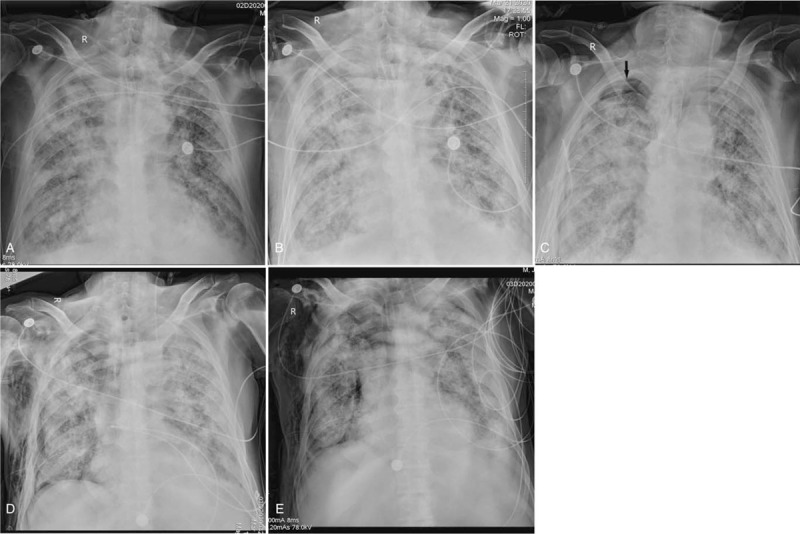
A 67-year-old male with 5 mobile chest X-rays before death. This series of X-rays showed the rapid progress of the lesions and the appearance of pneumothorax and subcutaneous emphysema. A. The first X-ray, extensive patchy opacities can be seen in both lung fields, involving the entire lung fields. B. Two days after the first X-ray, the second X-ray showed that patchy opacities increased in density and profusion. C. Three days after the second X-ray, the third X-ray showed that pneumothorax in the right upper lung field (arrow) appeared. D. Three days after the third X-ray, the fourth X-ray showed that the region of the right pneumothorax was enlarged, and the right subcutaneous emphysema appeared. E. One day after the fourth X-ray, the fifth X-ray showed that the region of the pneumothorax and subcutaneous emphysema was further enlarged. The patient died 1 day after the fifth X-ray.
